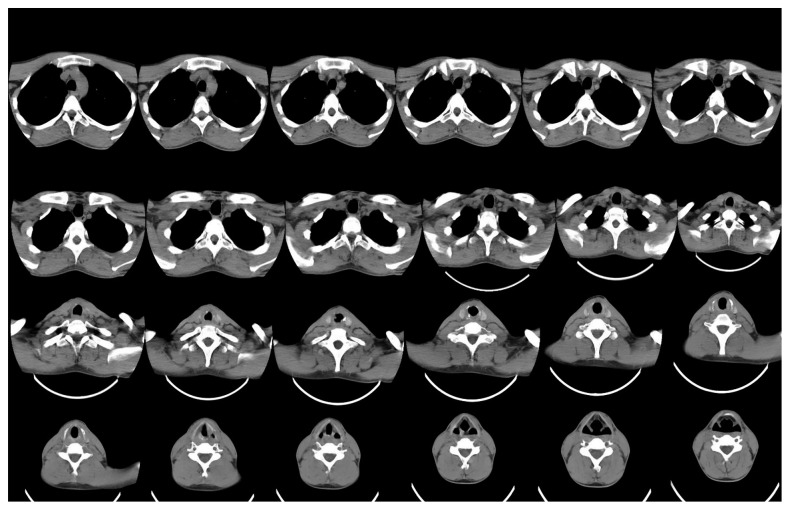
CT neck 6 month postoperatively.
